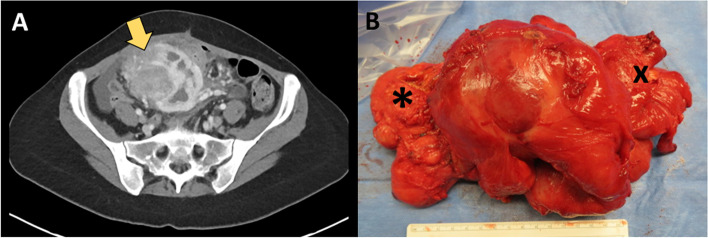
A Computed tomography image of intrabdominal mass (yellow arrow) and B en bloc resection of mass in relation to cecum (*) and small bowel (x)
